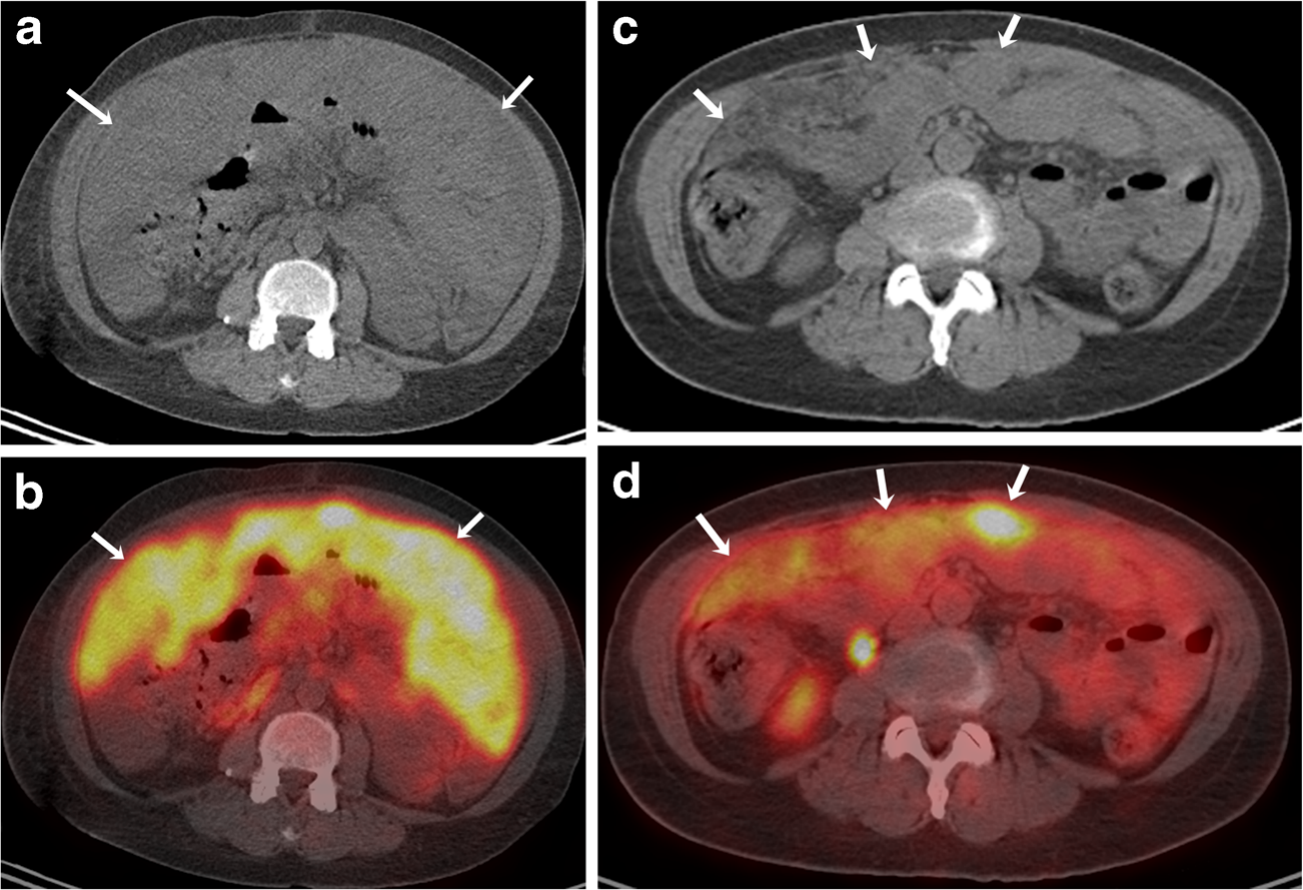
A 61-year-old female with vaginal melanoma and peritoneal metastasis. Axial unenhanced CT (a) and fused FDG-PET/CT (b) images show diffuse peritoneal thickening (arrows in a) with intense FDG uptake (arrows in b). Axial unenhanced CT (c) and fused FDG-PET/CT (d) images after treatment with imatinib shows a significant decrease in peritoneal thickening (arrows in c) and FDG uptake (arrows in d)
